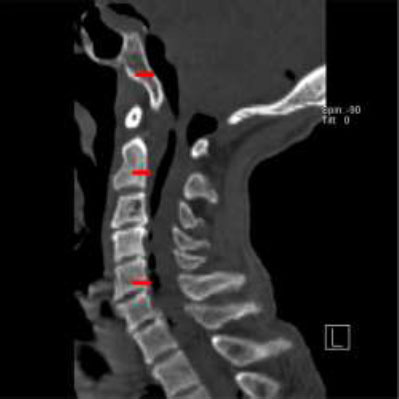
Air densities visible in the cervical CT scan extending from the clivus to thoracic vertebrae (pneumorrhachis), indicative of subarachnoid pleural fistula.
